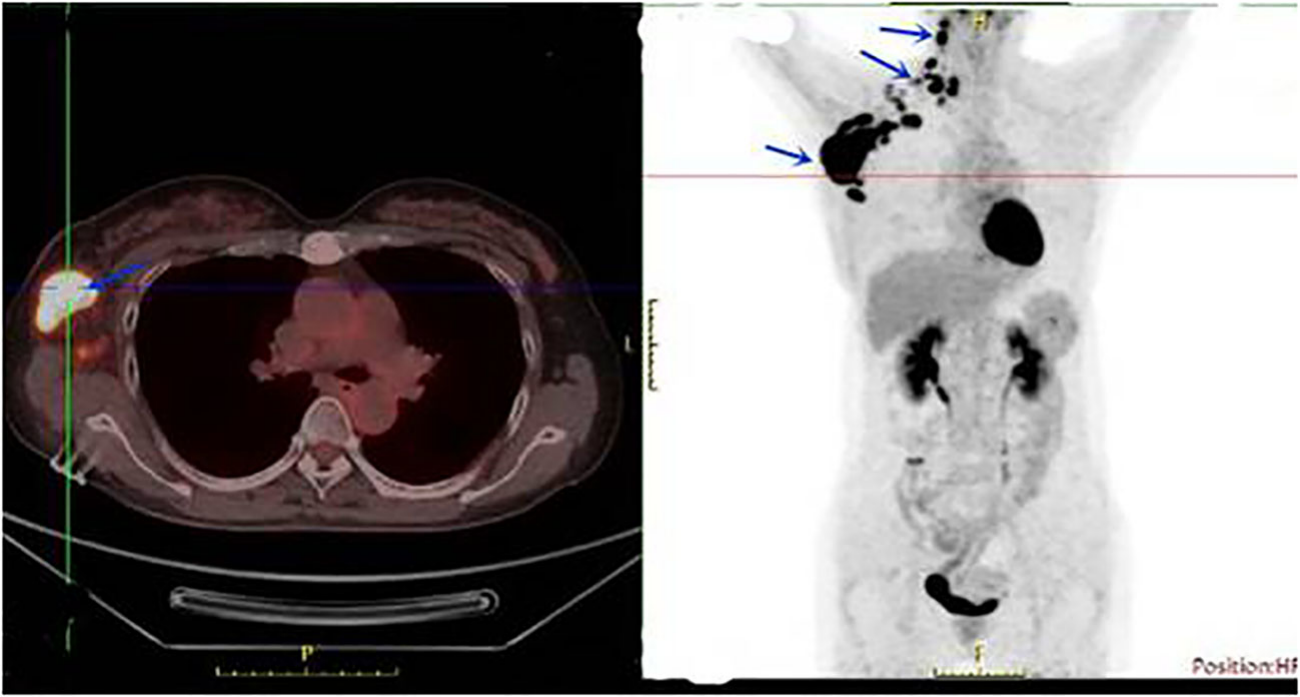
PET-CT showed multiple masses in the right axilla, right supraclavicular region, and spleen in March 2020.
